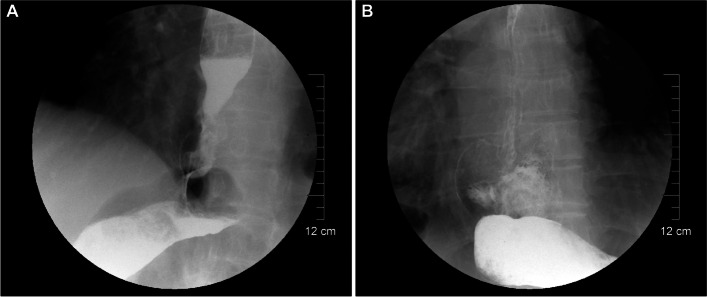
Iohexol contrast radiography performed 30 days after surgery. A Contrast passed through the anastomosis smoothly without leakage or stenosis. B Outline of the remnant stomach with a pseudofornix
